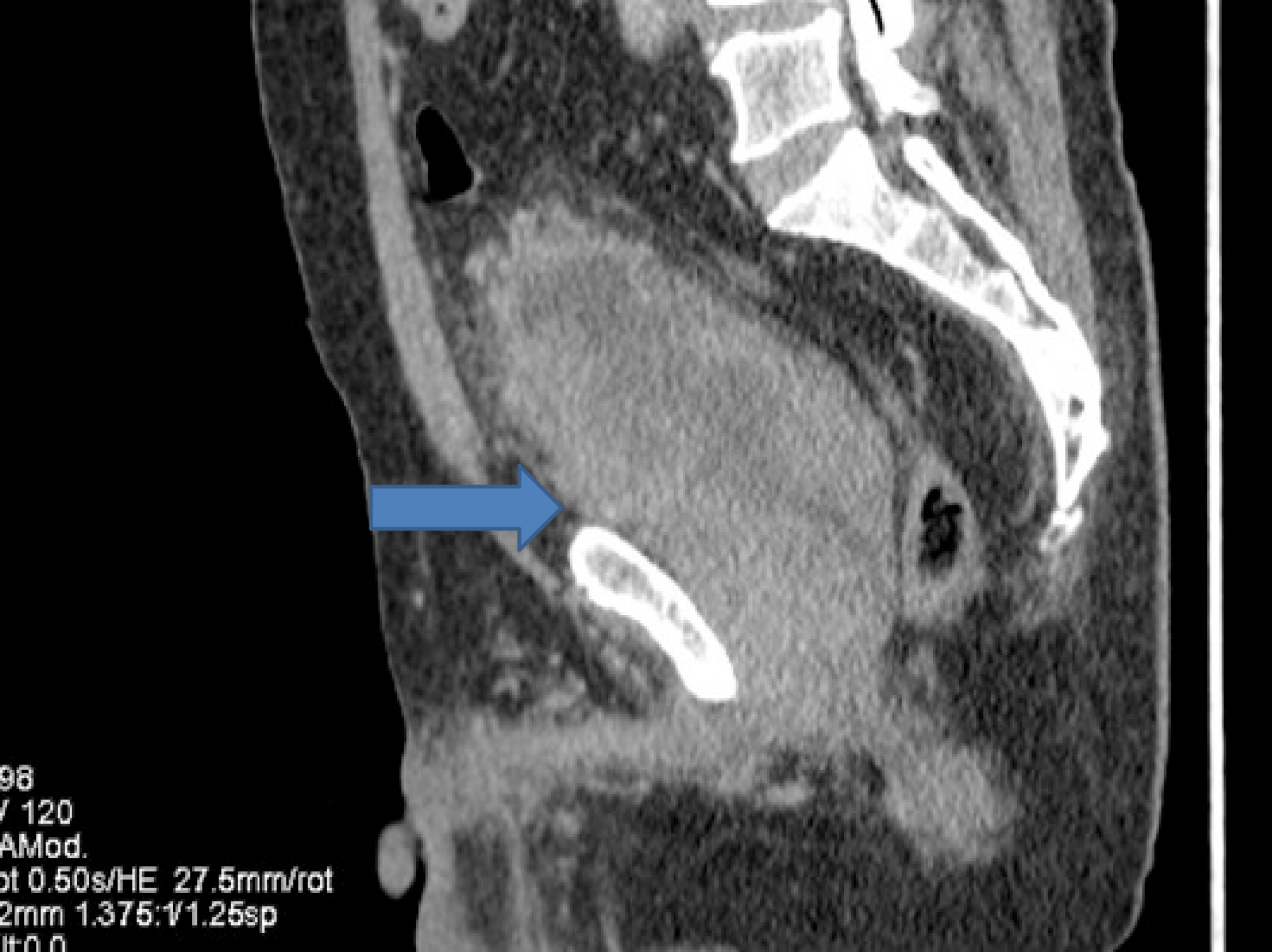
Computed tomography (CT) showing progression in urinary bladder.
